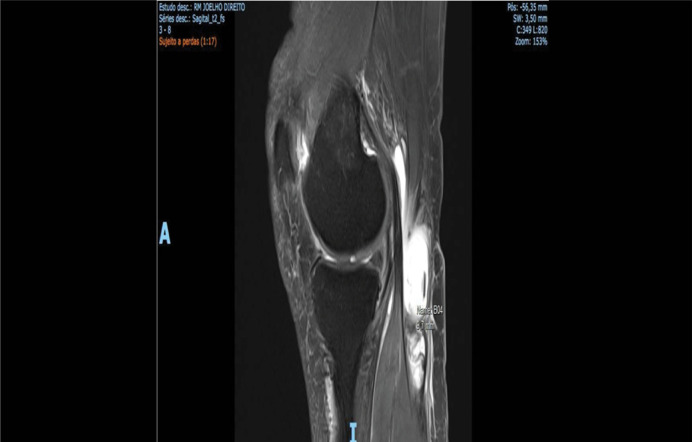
Sagittal magnetic resonance image demonstrating a partially ruptured Baker's cyst in its distal part.
